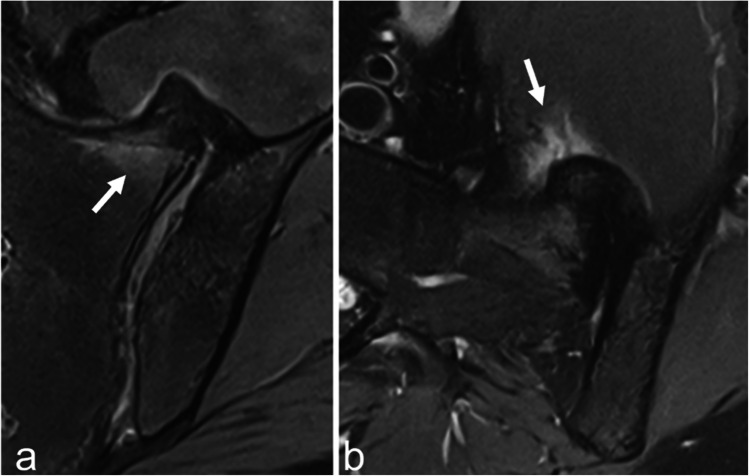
A 68-year-old man with hyperostosis of the SIJ. Slight BME at the base of the bridge (arrow) and in the adjacent soft tissues (arrow) on coronal oblique (a) and axial oblique (b) FS T2-weighted images
